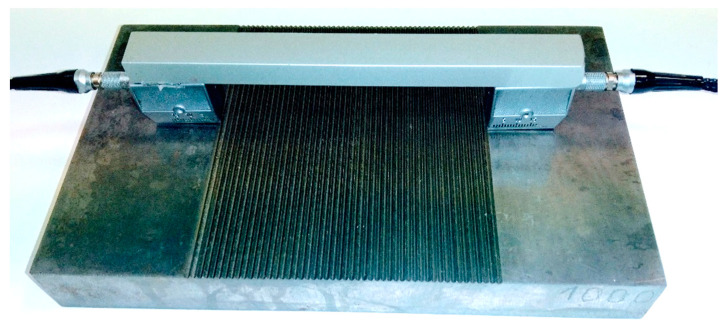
Ultrasonic probes used in research.
